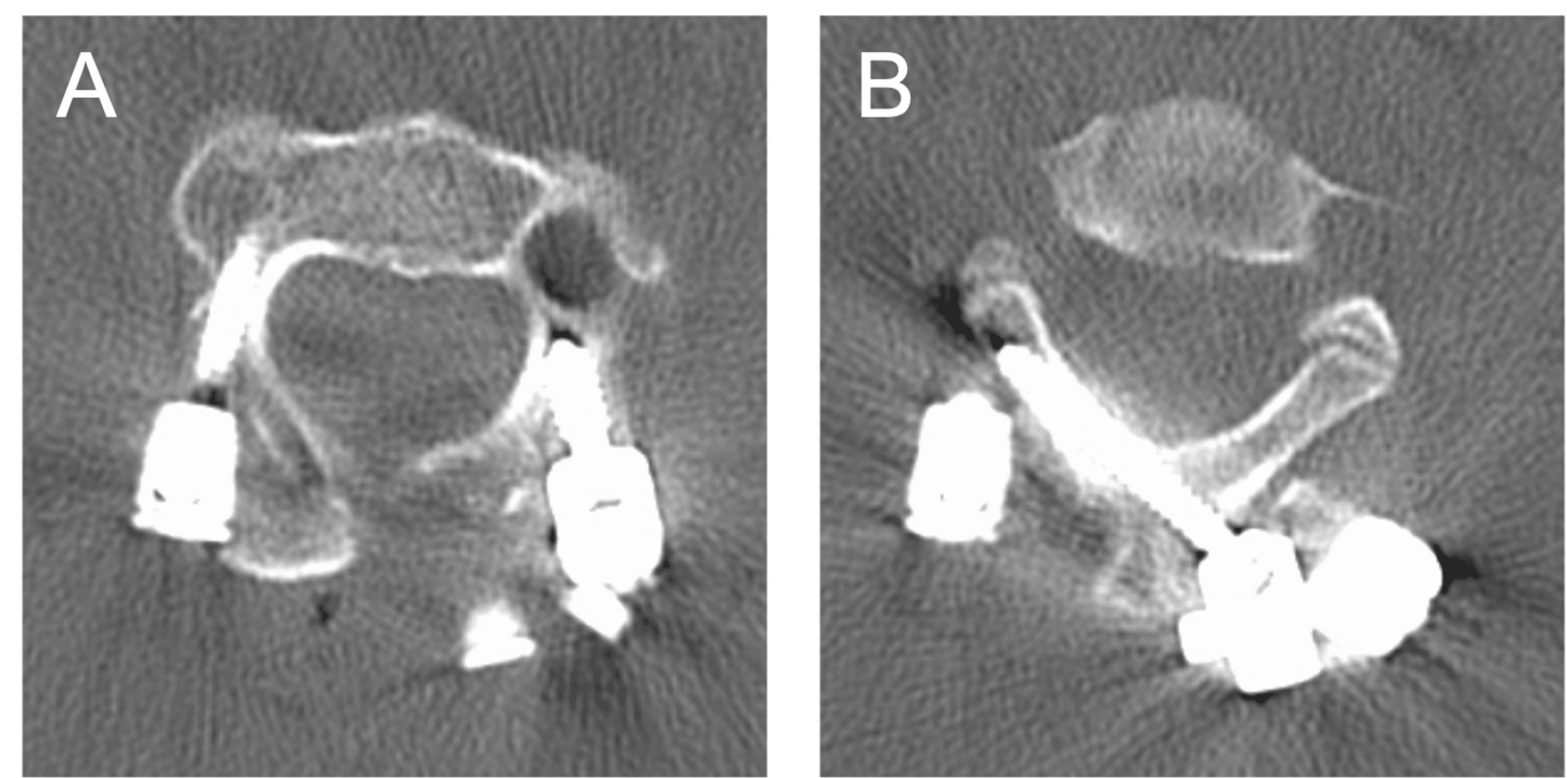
Postoperative CT Axial CT images at slightly different C2 levels (A, B) demonstrate satisfactory screw trajectories: panel A (more cranial) shows the large-diameter left C2 pars screw together with the contralateral C2 pedicle screw, whereas panel B (more caudal) shows the left C2 intralaminar screw.
